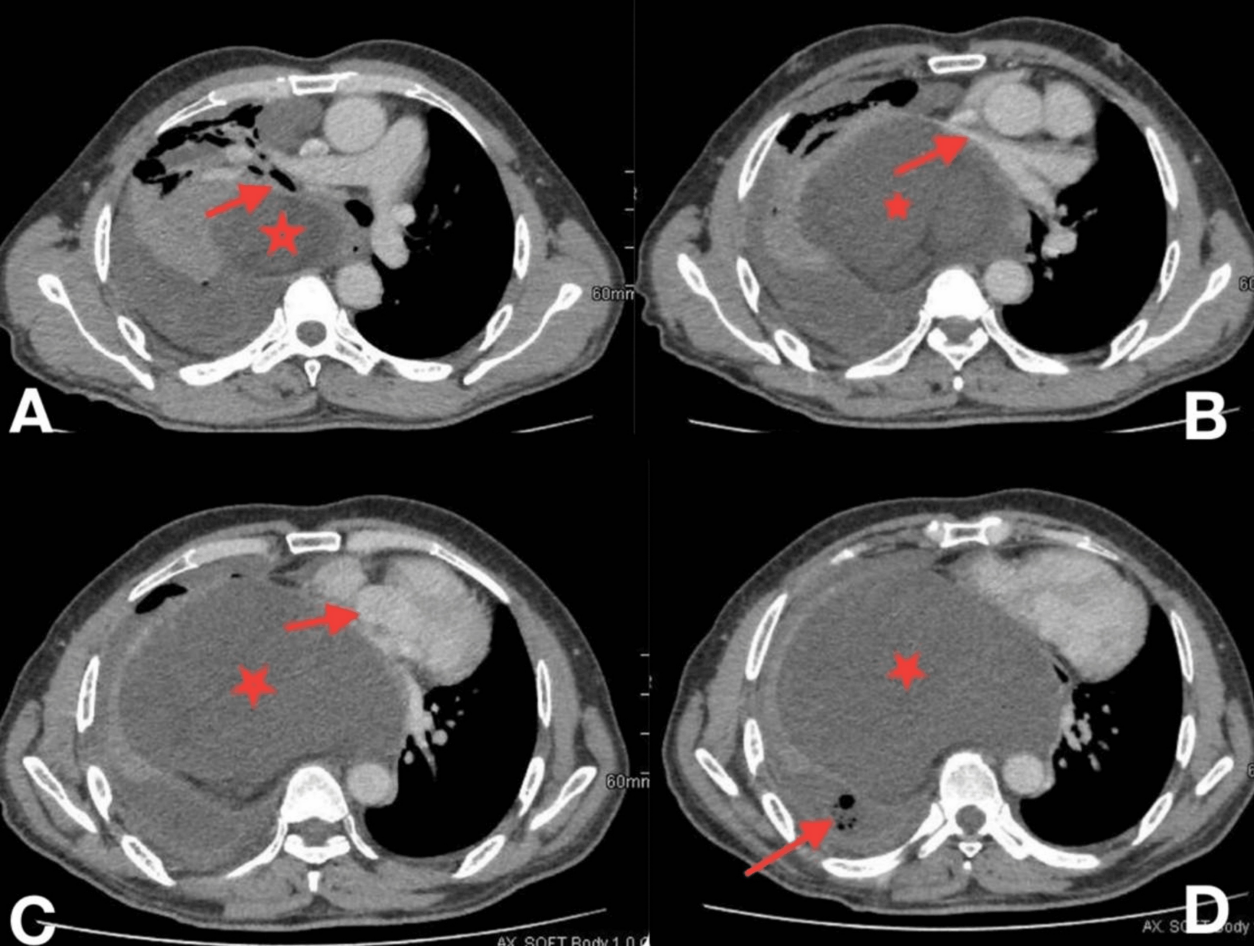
Enhanced axial chest CT scan soft tissue window shows a large mass (star) extended to the mediastinum causing compression to the right main bronchus (arrow) (A), with a significant displacement of the cardiovascular mediastinal structures toward the left side with an attenuation of the lumen of pulmonary veins (arrow) (B), left and right atrium (arrow) (C), and multiloculated right pleural effusion with thick irregular pleural enhancement and multiple septations associated with gas bubbles (arrow) (abscess) (D). CT: computed tomography
